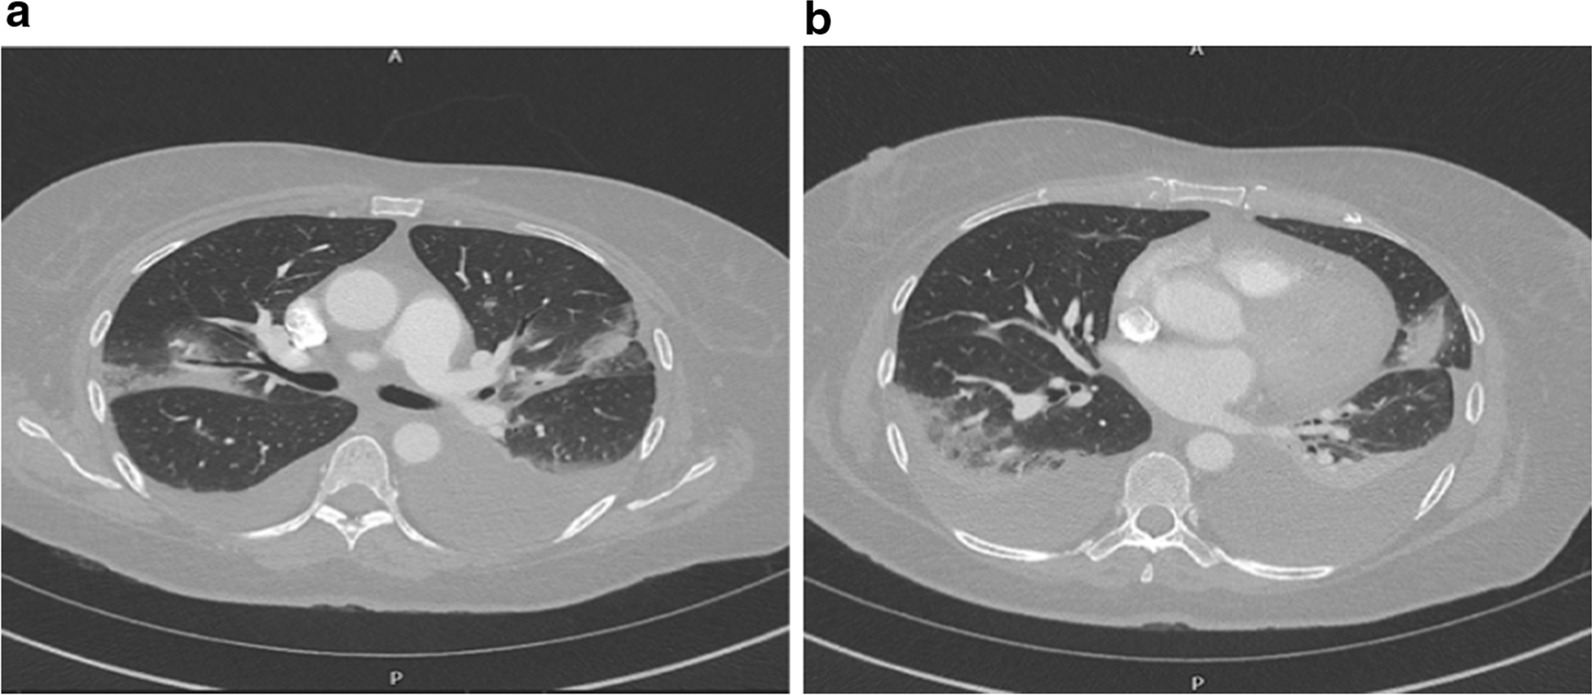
a Computed tomography chest scan showing bilateral consolidation. b Computed tomography chest scan showing bilateral consildation and large bilateral pleural effusions
